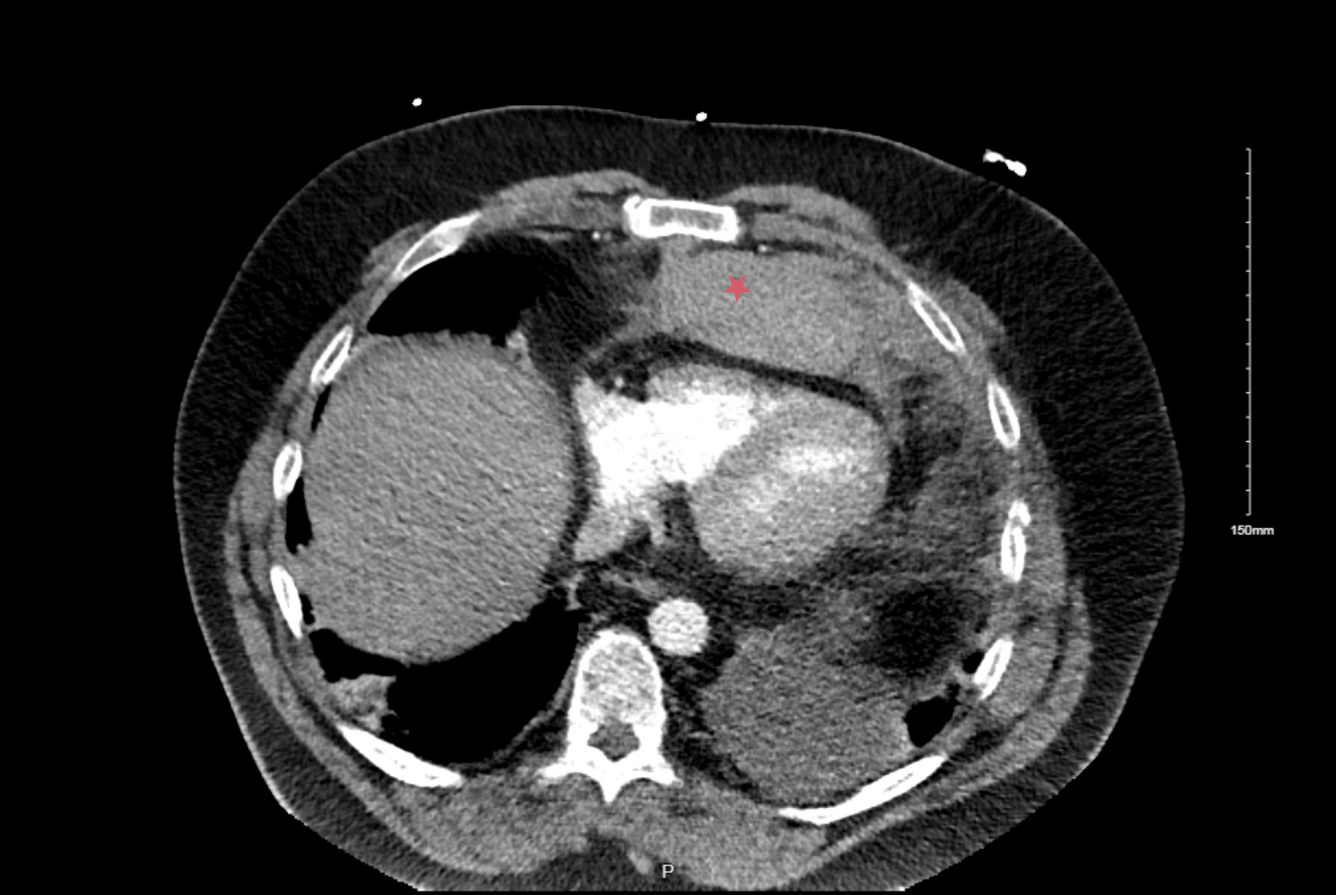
Axial view of the computed tomography pulmonary angiogram revealing a large anterior hematoma (red star)
